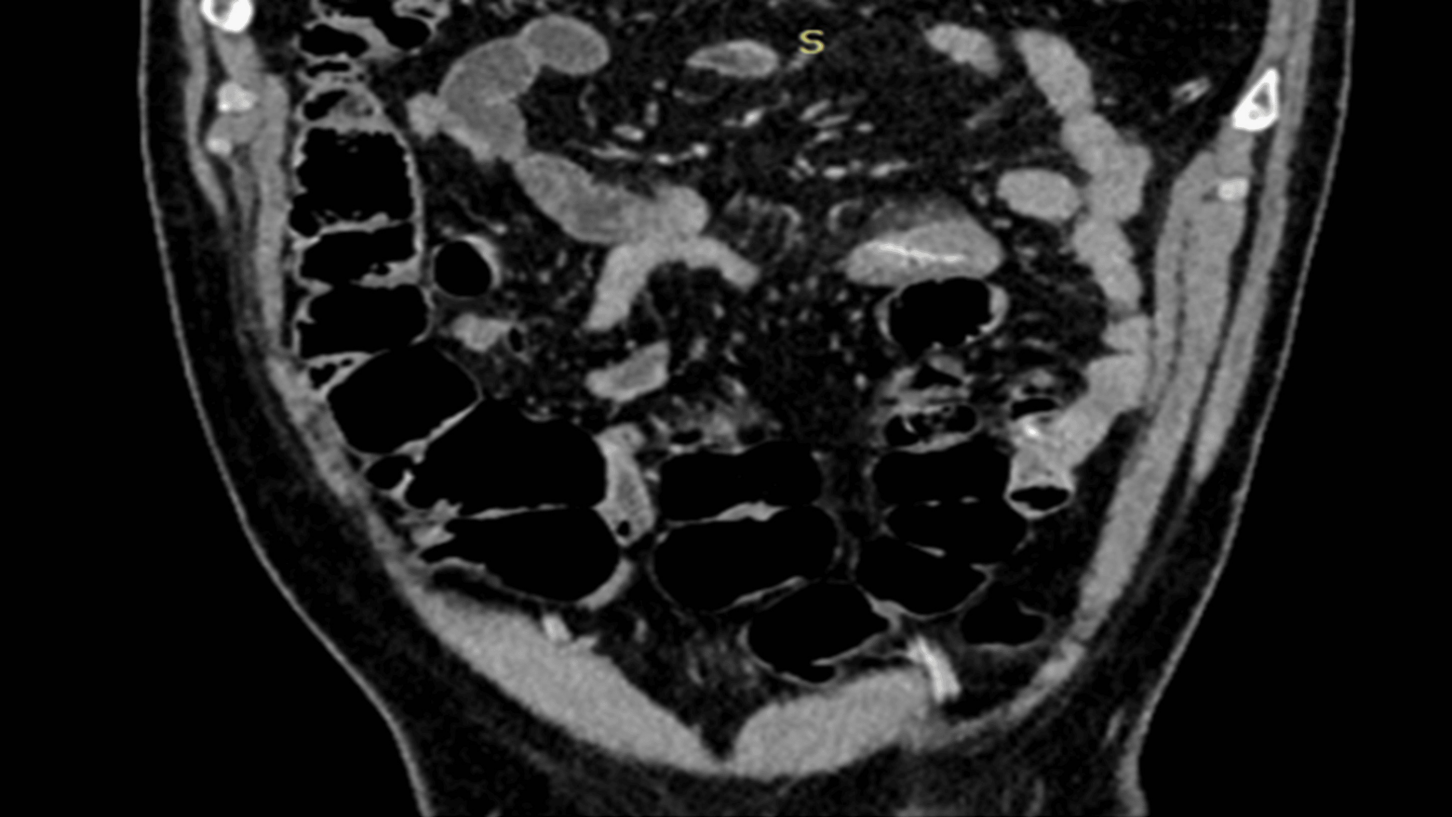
Contrast-enhanced computed tomography scan of the abdomen and pelvis showing a linear foreign body in the proximal jejunum (arrow) with surrounding bowel wall thickening and mesenteric fat stranding
